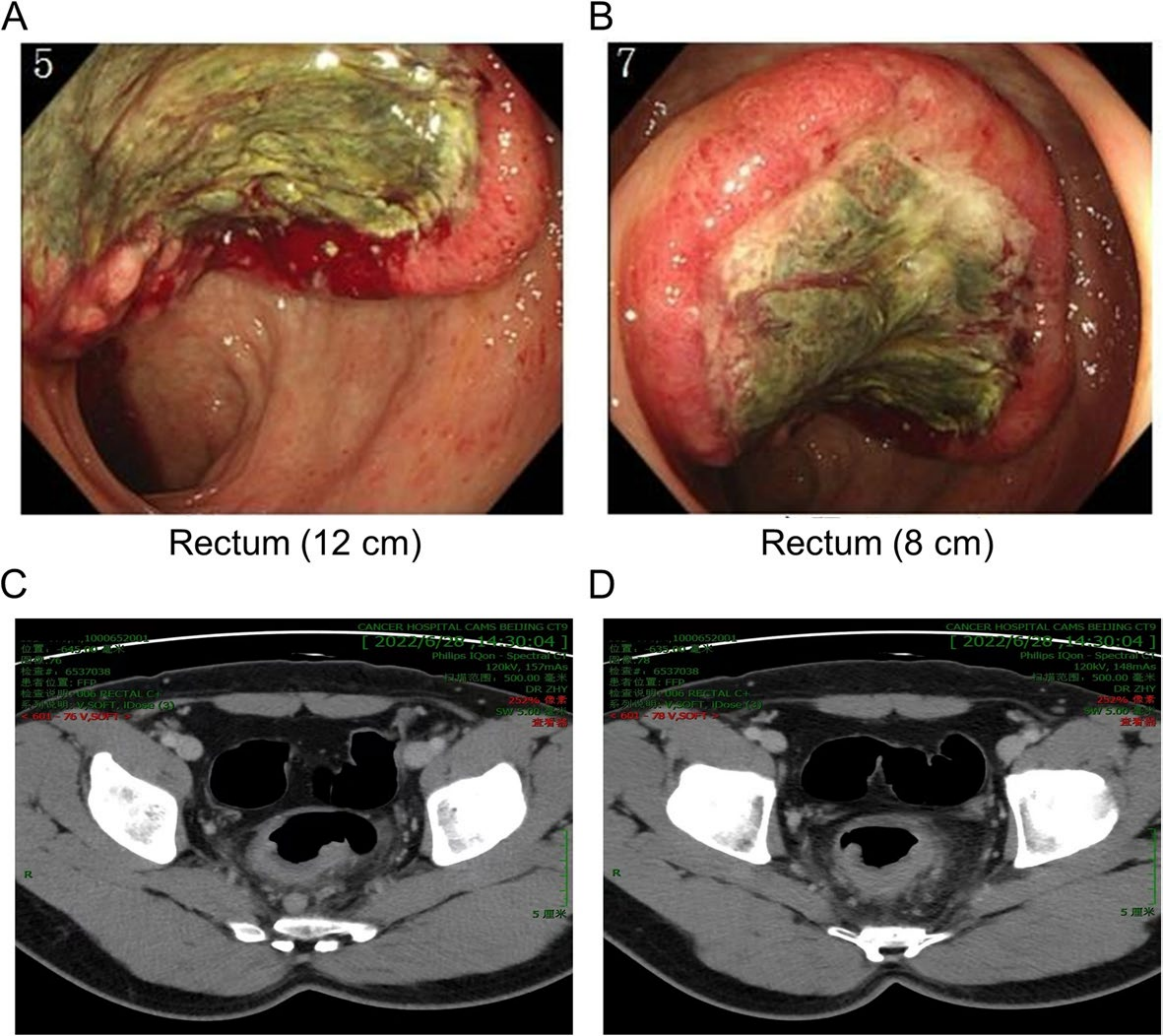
Clinical findings. A, B Colonoscopy revealed an ulcerated and irregular mass approximately 8-12 cm from the anus with obvious lumen stenosis. C, D Computed tomography (CT) shows thickened walls of the sigmoid and upper rectum. Multiple regional lymph nodes showed strengthening signals
